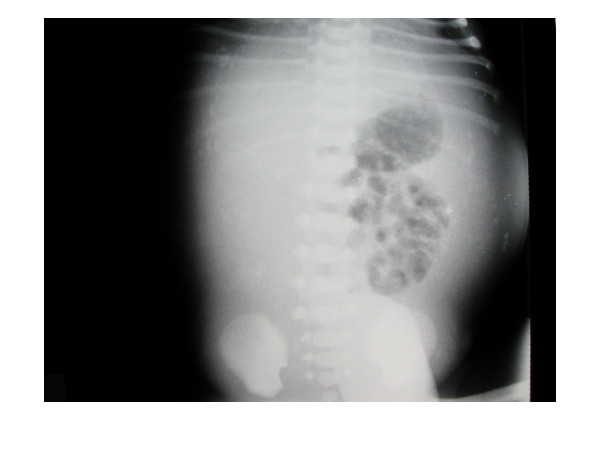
Abdominal radiograph taken 1 hour after birth, shows mottled calcification in the right upper quadrant, curvilinear calcification in the left flank and several loops of non-dilated bowel, but no free gas.
